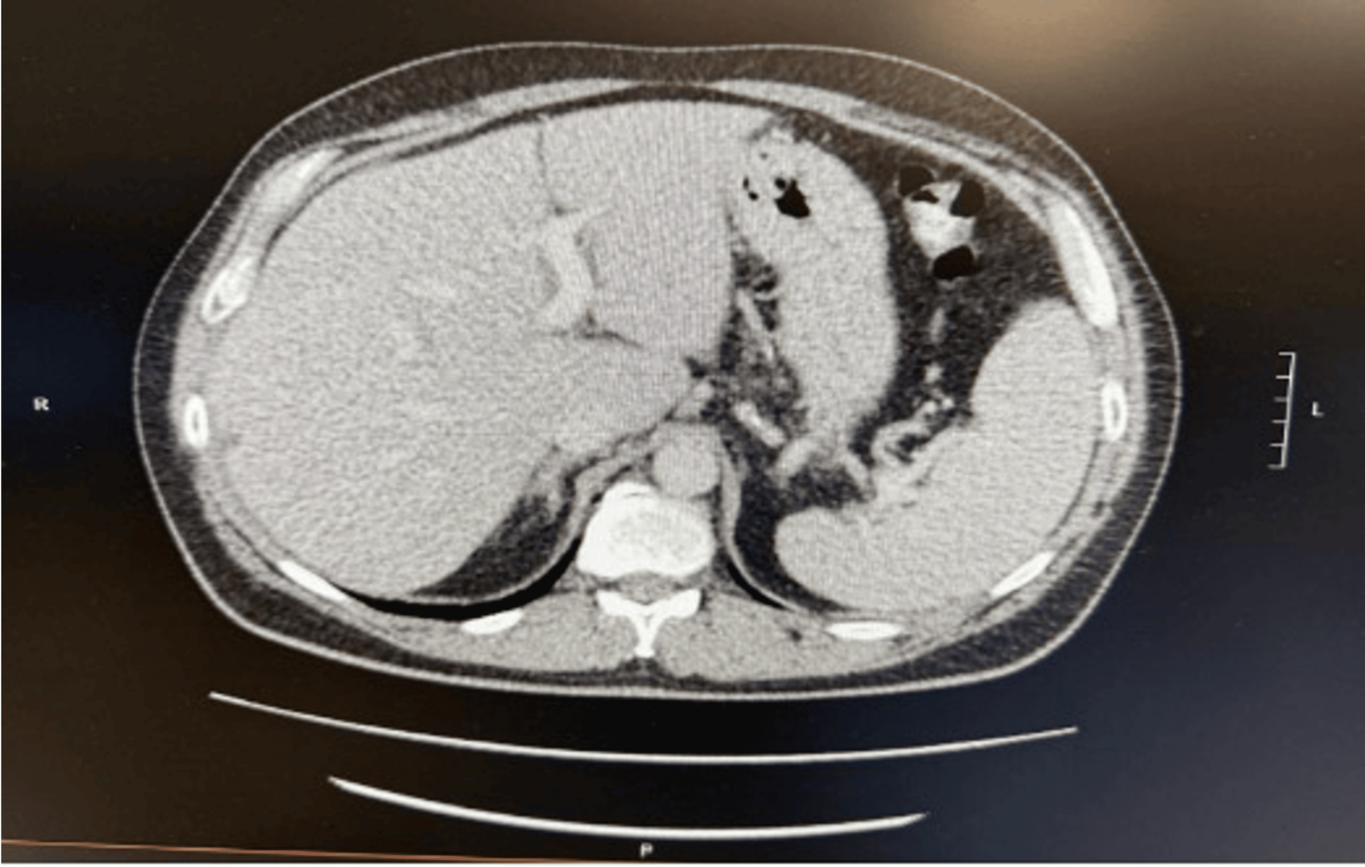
High-resolution contrast-enhanced CT scan of the abdomen (axial view) showing normal liver, gallbladder, pancreas, and spleen.
